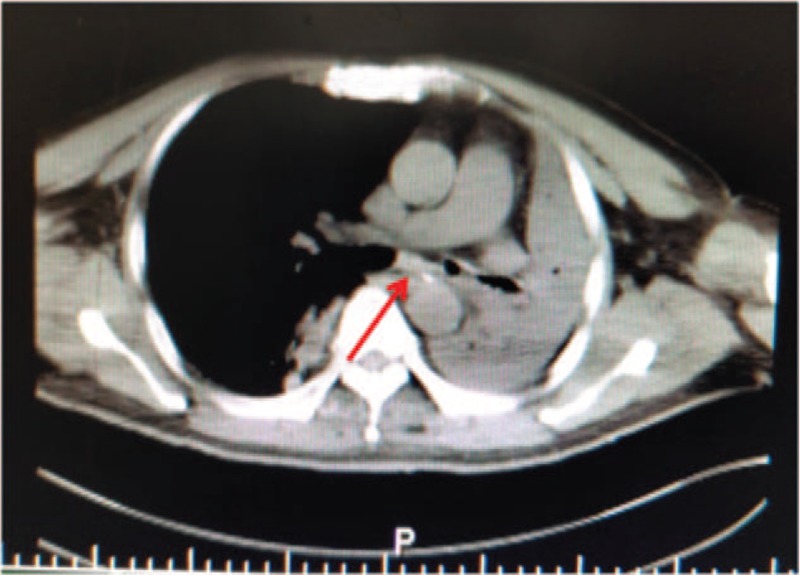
CT showed pulmonary atelectasis of the left lung and the possible of a FB in the left main bronchus.
